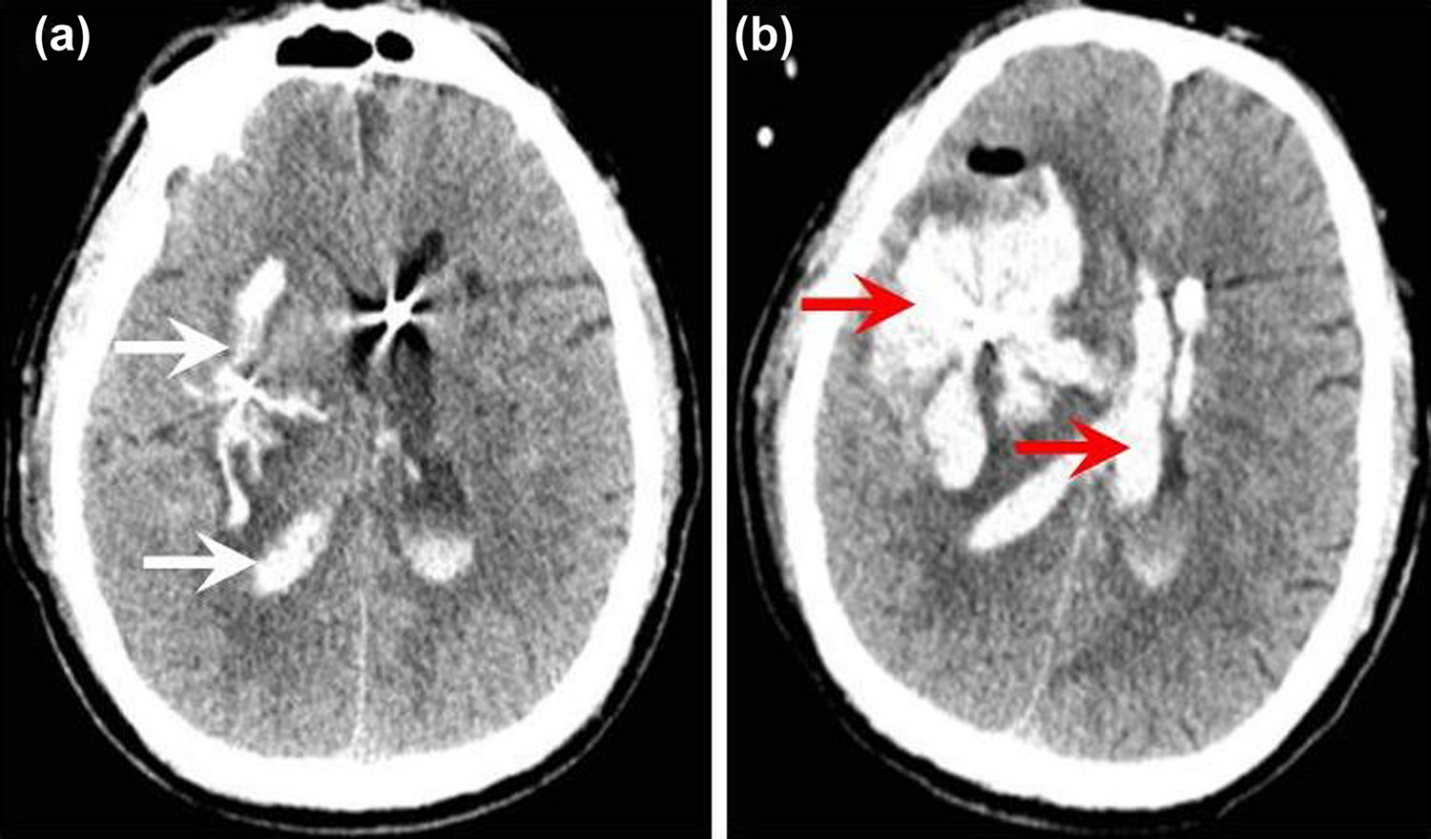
Postoperative intracranial rebleeding in patients underwent surgery. (a) A small amount of residual hematoma in the brain and the ventricles were observed on postoperative CT follow-up (the white arrowhead). (b) The intracerebral hemorrhage and the intraventricular hemorrhage increased significantly (the red arrowhead) compared with the previous follow-up CT on which the residual hematoma volume was very small (a). A significant increase in midline shift has also been noted.
